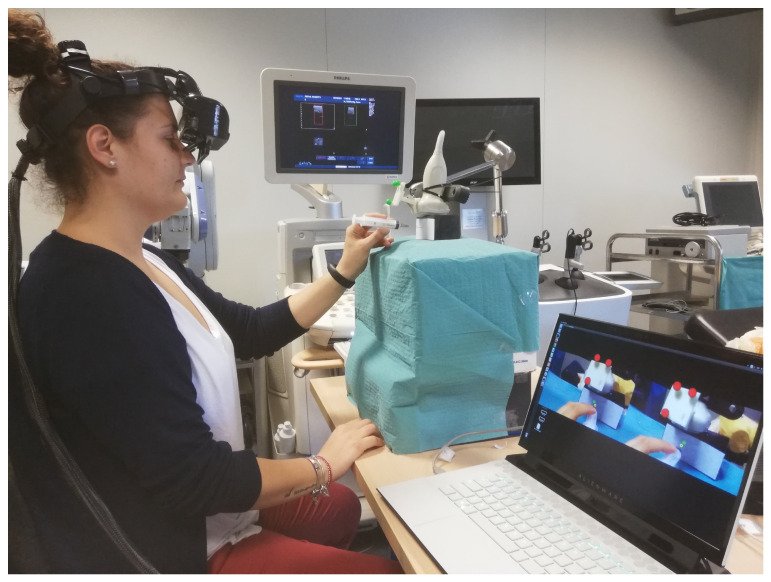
Experimental setup with a user while performing the biopsy task. In the lower right corner are the augmented images projected onto the displays, whereas, on the Philips machine monitor, the real-time ultrasound acquisition can be seen.
